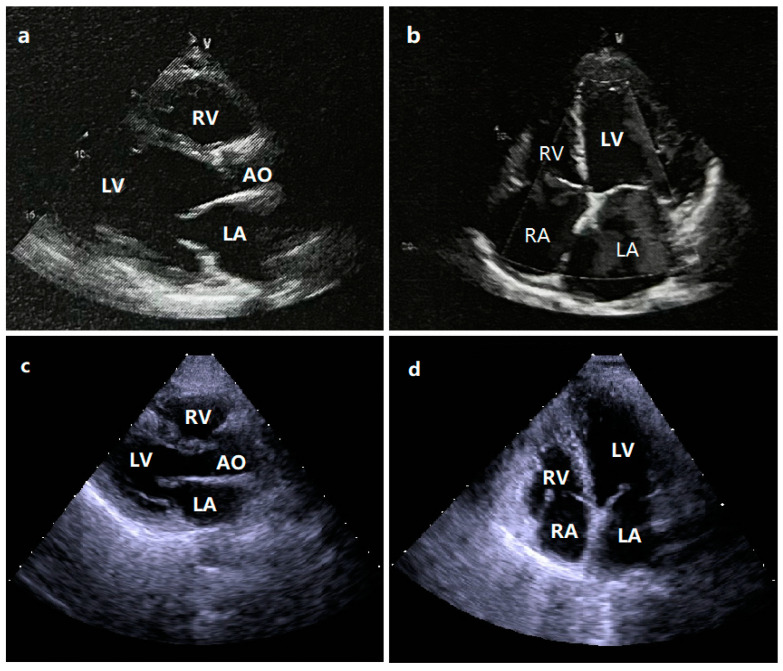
The representative transthoracic two-dimensional echocardiographic images from the proband affected with dilated cardiomyopathy and a healthy individual as a control: (a) transthoracic echocardiography findings at the left ventricular longitudinal view from the proband; (b) transthoracic echocardiography findings at the apical four-chamber view from the proband; (c) transthoracic echocardiography findings at the left ventricular longitudinal view from the control; (d) transthoracic echocardiography findings at the apical four-chamber view from the control. The echocardiographic images denote severe left ventricular dilation and systolic dysfunction. LV, left ventricle; RV, right ventricle; LA, left atrium; RA, right atrium; AO, aorta.
